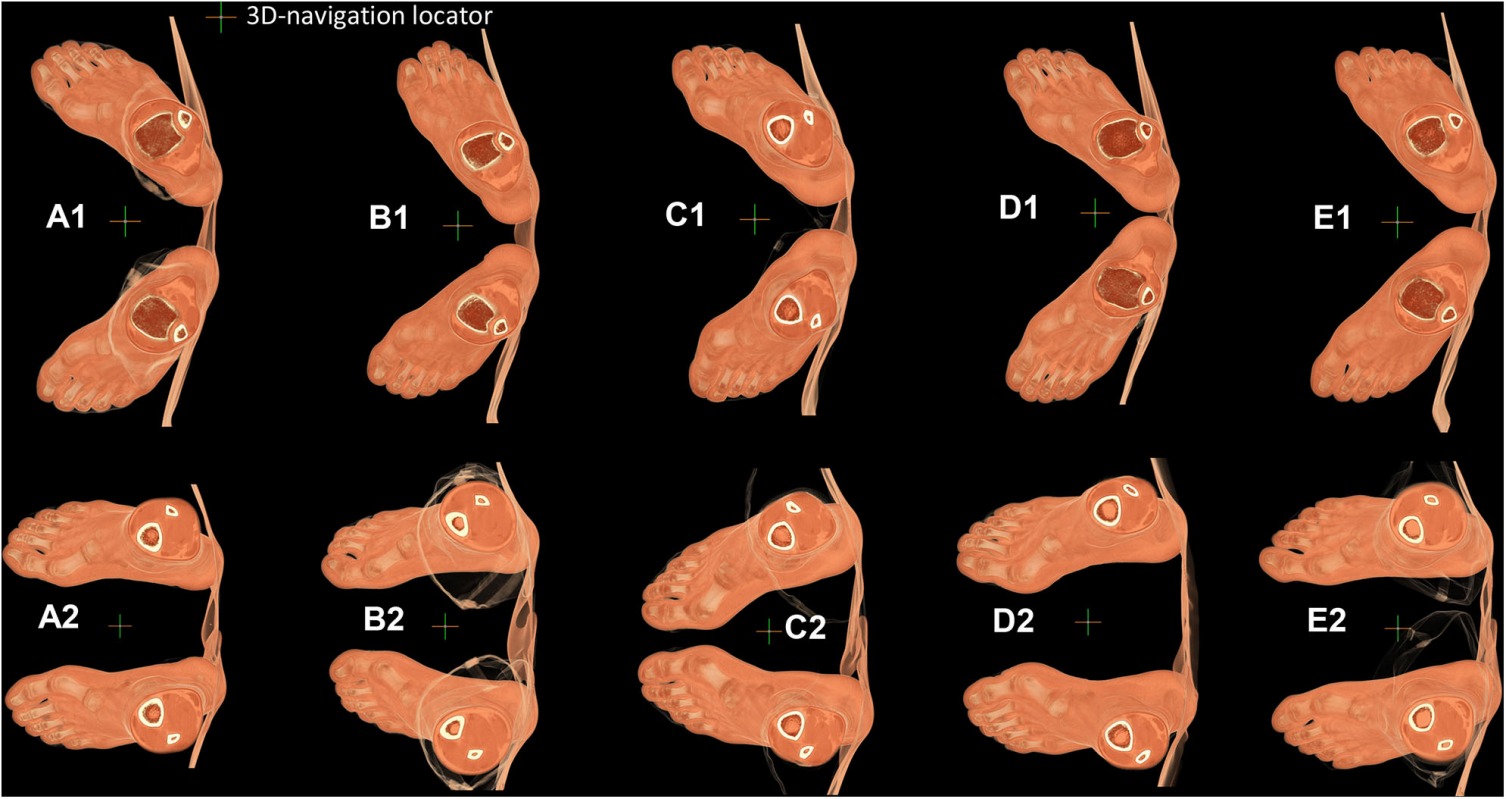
Each participant's posture of both feet obtained by 64-slice CT scanner. (A–E). Feet of P1, P2, P3, P4, P5, respectively. (A1–E1). Foot posture from the first time scan. (A2–E2). Foot posture from the second time scan.
